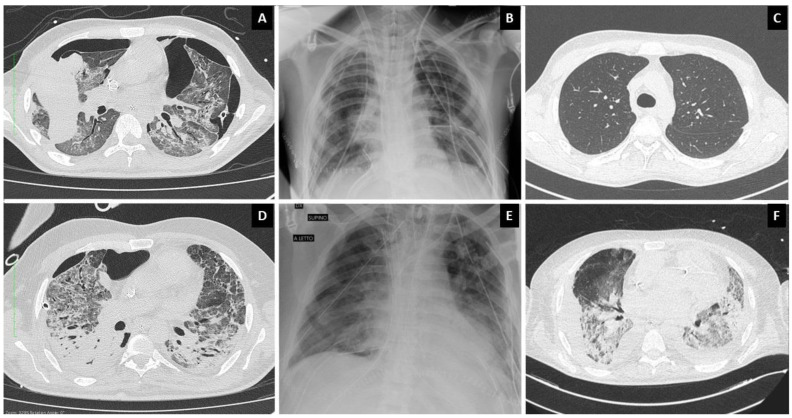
Patients’ imaging. (A) Patient-1 preoperative CT; (B) patient-1 chest X-ray on post-operative day 1; (C) patient-1 CT 4 months after transplantation; (D) patient-2 preoperative CT; (E) patient-2 chest X-ray on post-operative day 1; (F) patient-2 CT before death.
